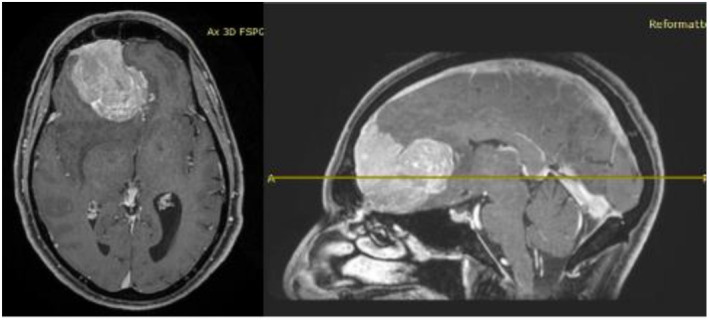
Pre-operative brain MRI showing the anterior cranial fossa meningioma with perilesional oedema.
